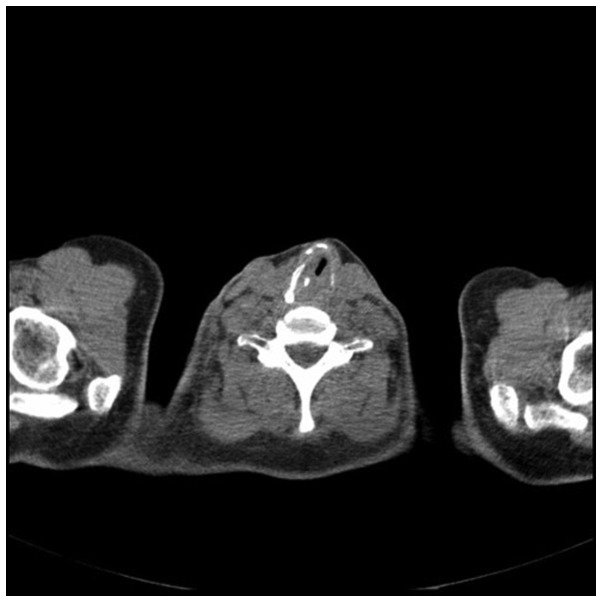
Postoperative computed tomography scan following resection of the tracheal tumor.
